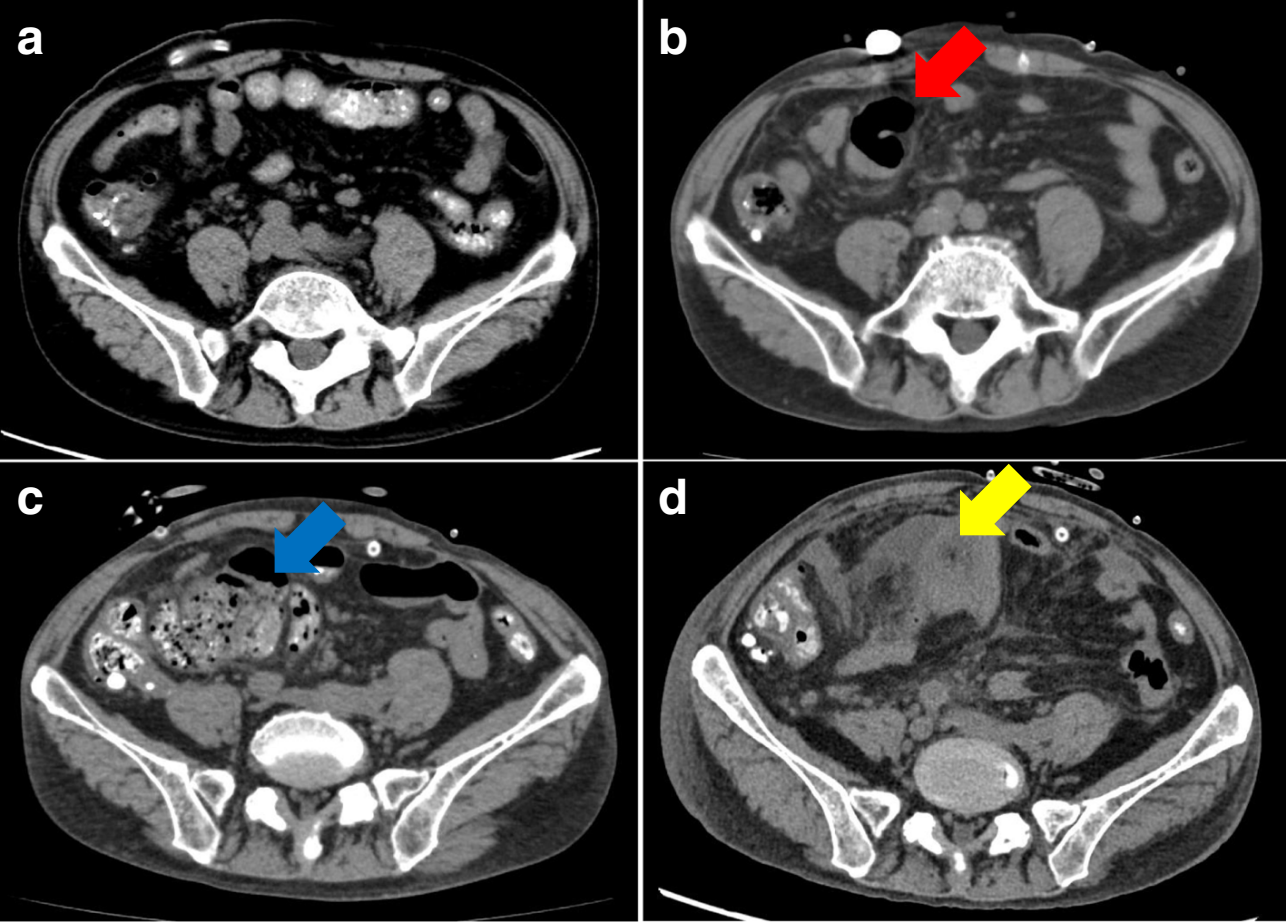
Longitudinal abdominal computed tomography images. a Bowel adhesion had not been noted 5 years previously. b Local adhesions encapsulating the intestine (red arrow) are detected after an episode of peritoneal dialysis-associated peritonitis 1 year previously. c Localized dilation of the intestine, suggesting adhesive bowel obstruction (blue arrow), is noted on admission. d On hospital day 23, it is seen that the intestinal contents disappeared and the dilated intestine collapsed (yellow arrow), indicating that the intestinal contents had leaked into the abdominal cavity
